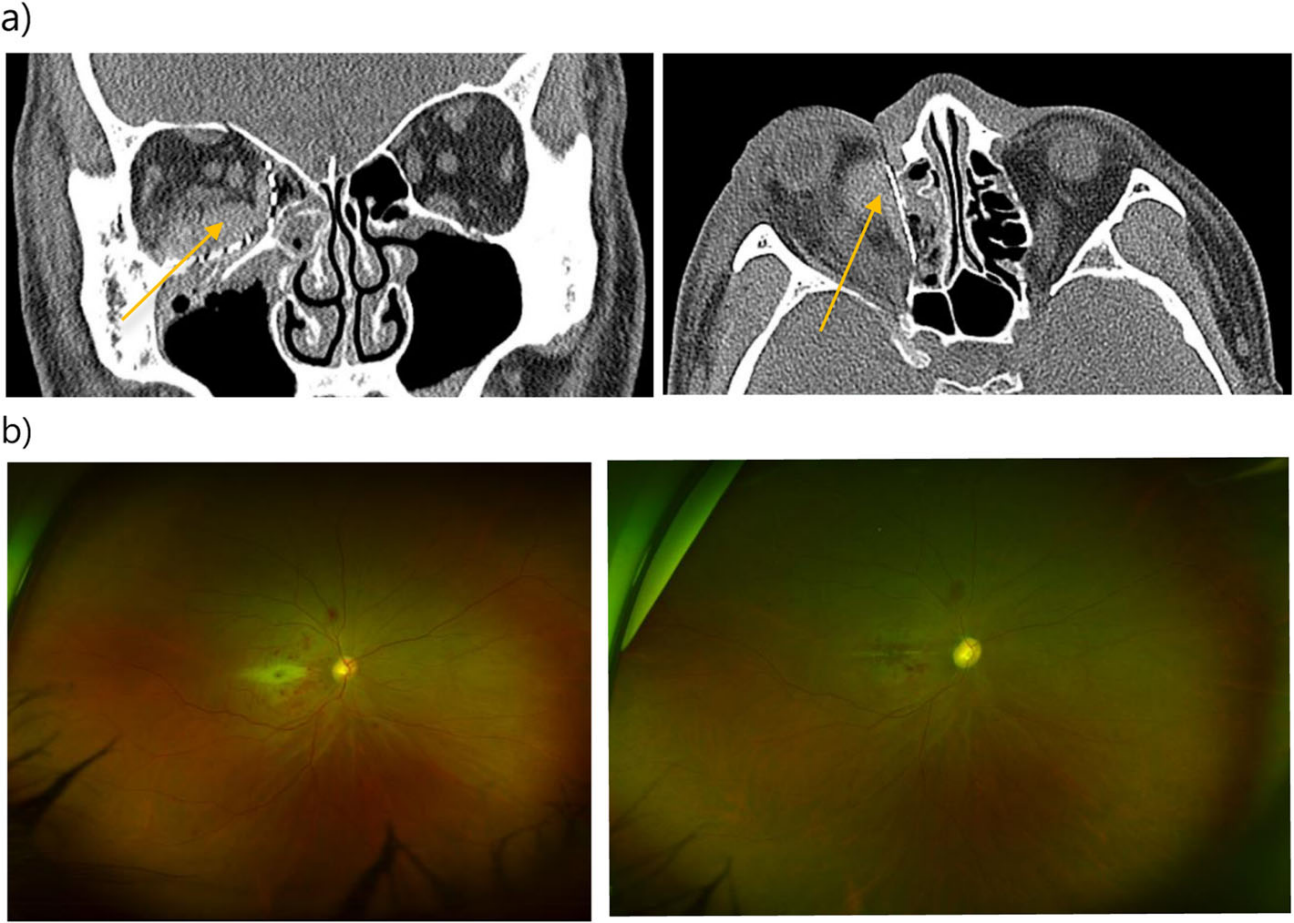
Postoperative CT images and fundus photographs. a Postoperative CT images of coronal and axial sections. The right medial and inferior orbital walls were reconstructed, and the med-por was properly located. However, a soft tissue density lesion was founded in left extraconal space along the medial and inferior orbital wall, indicating a retrobulbar hematoma (yellow arrow). b Left: fundus photograph of the right eye showing a cherry-red spot, a milky-white lesion on macula, and mild venous dilation. Right: 1-month after the previous fundus photograph
